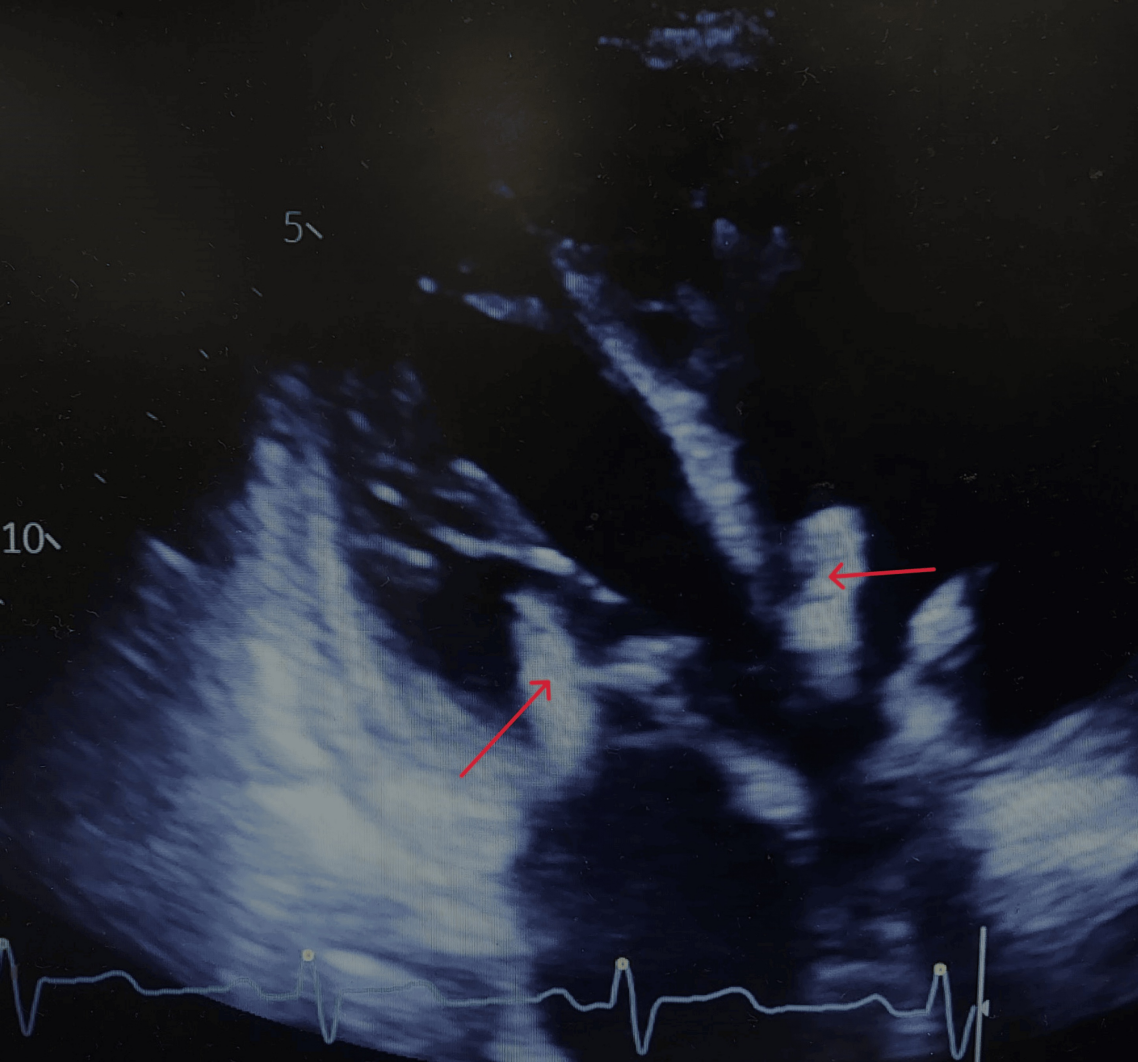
TTE showing the apical four-chamber view, long axis, and left- and right-sided infective endocarditis with mobile vegetations. TTE: transthoracic echocardiography
